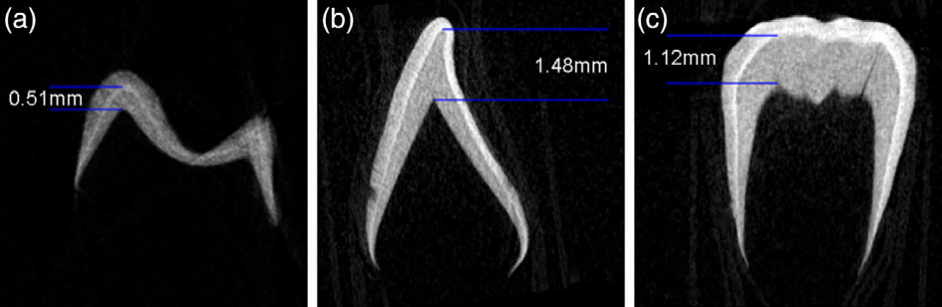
Micro‐CT images slices of upper second deciduous molar, coronal view (a), and coronal view (b) and sagittal view (c) of lower left first deciduous incisor from individual 469 from the stack collection (Royal College of surgeons England) aged 40.5 weeks gestation. Measurements in mm are shown of the depth of dentine developed in the cuspal (a) and incisal (b and c) areas [Color figure can be viewed at http://wileyonlinelibrary.com]
